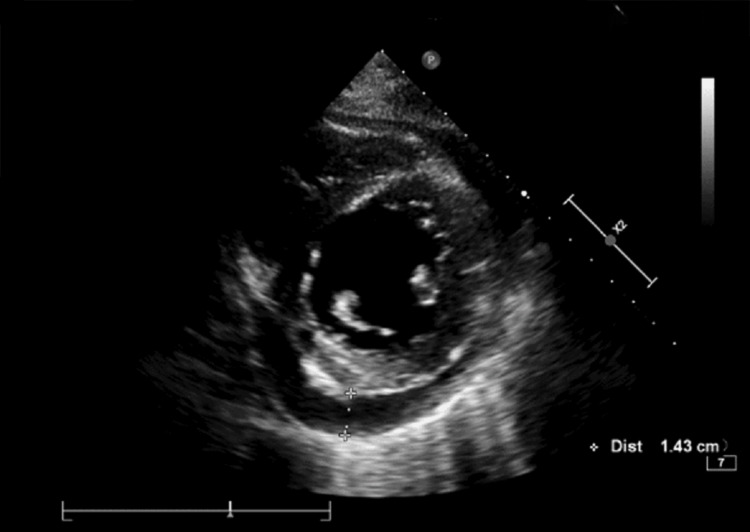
Pericardial effusion visualized on transthoracic echocardiography. Findings of moderate pericardial effusion measuring up to 14 mm overlying the right heart chambers, with no evidence of tamponade.
